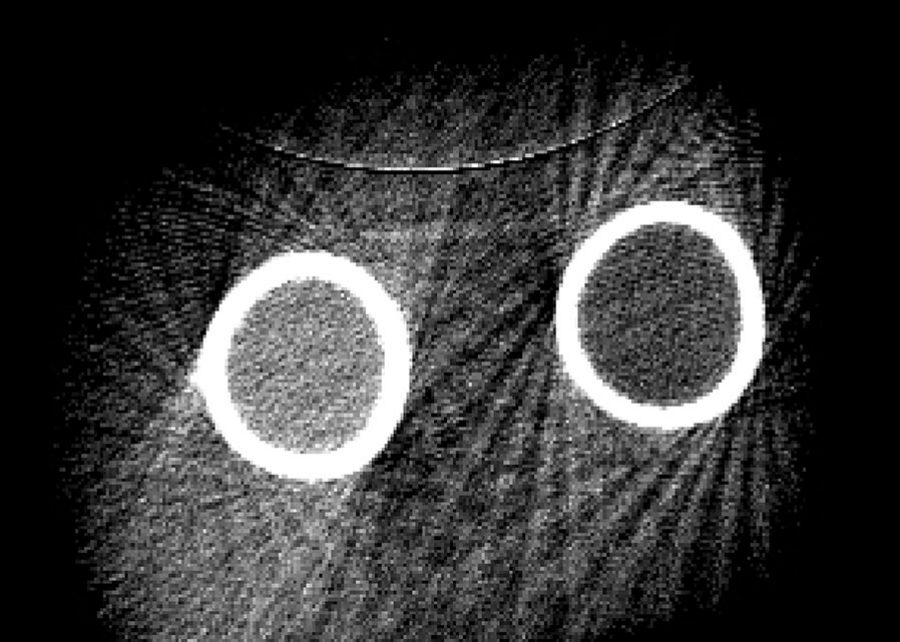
The scanned QRM-EFP images obtained from peripheral CBCT with 130 kvp, 10 mA and 20 ms exposure time
